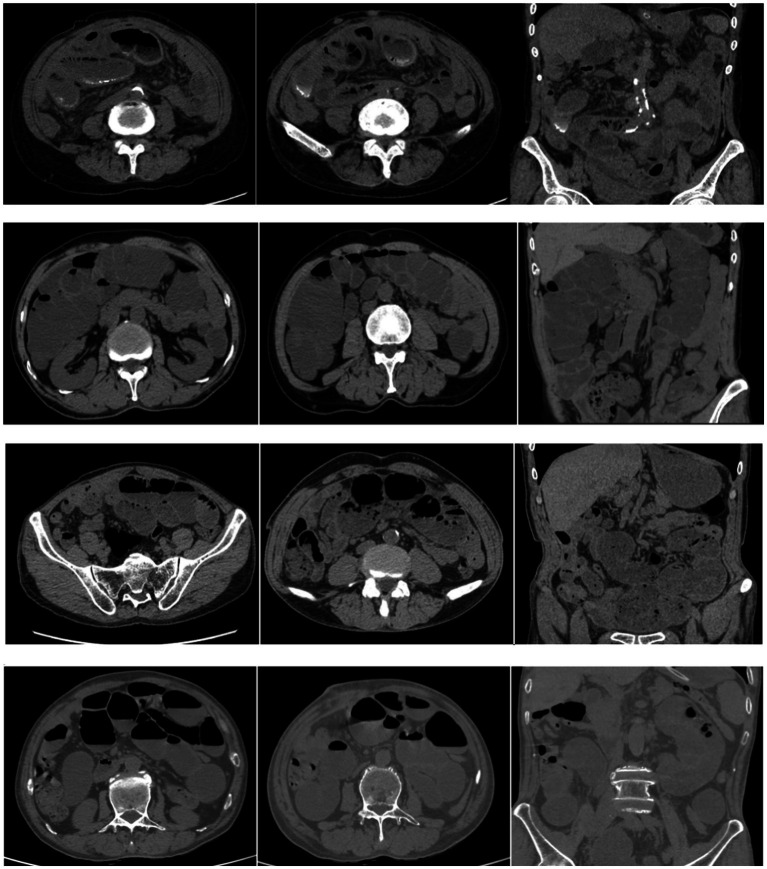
CT images of patients with abdominal cocoon syndrome.
